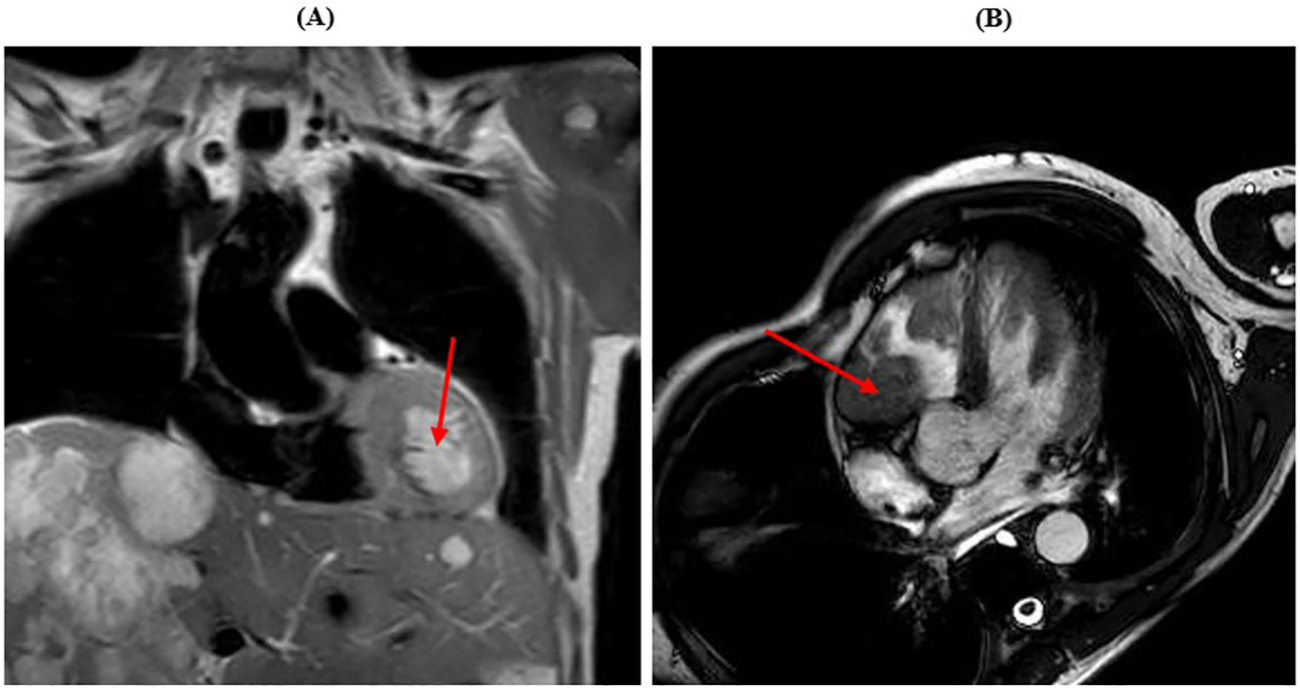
Cardiac MRI showing myocardial metastases. (A) The red arrow shows the lesion in the left ventricle. (B) The red arrow shows the lesion in the right ventricle.
